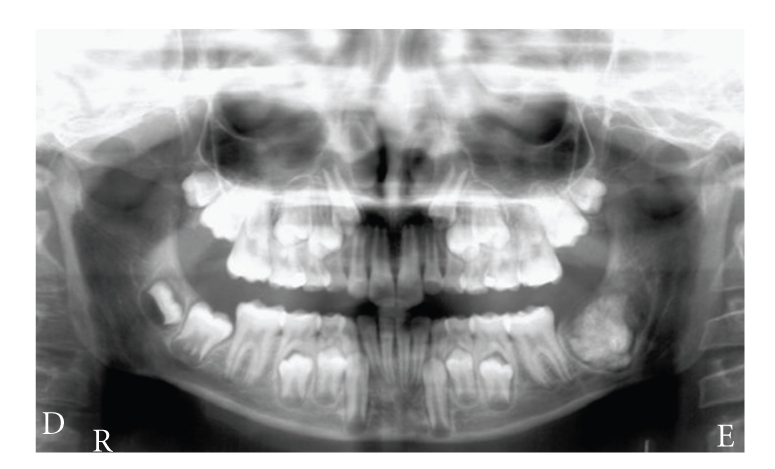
Initial panoramic radiography showing well-defined radiolucent region, which contained an irregular radiopaque mass measuring 3 cm in diameter. This lesion occupied a zone from the lower left second molar area to the left ramus.
